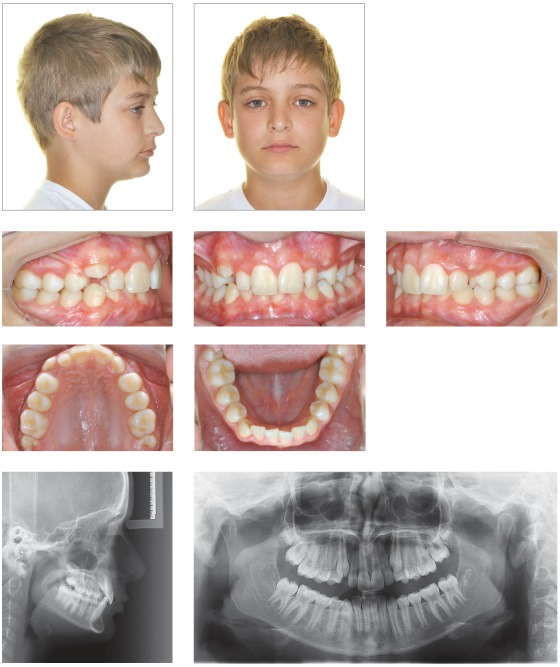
Initial records: extra- and intraoral photographs, lateral cephalogram and panoramic radiograph.
